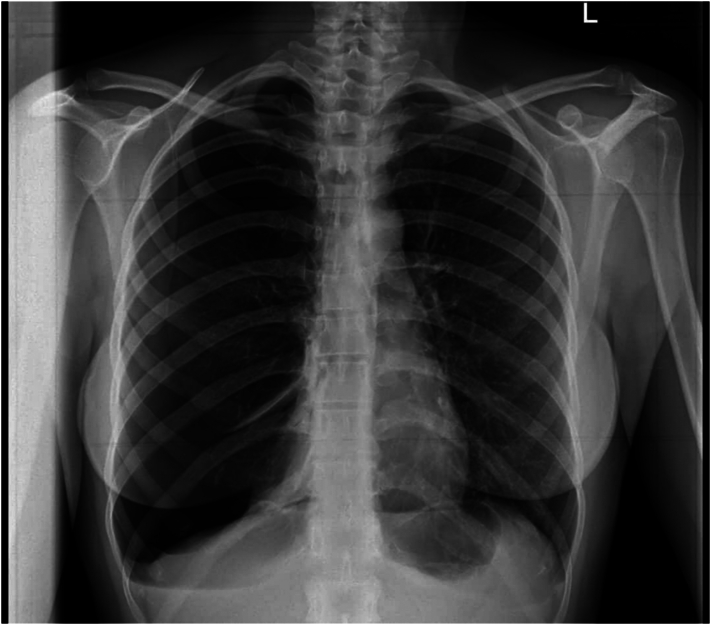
Chest X-Ray showed pneumothorax on the right side.
